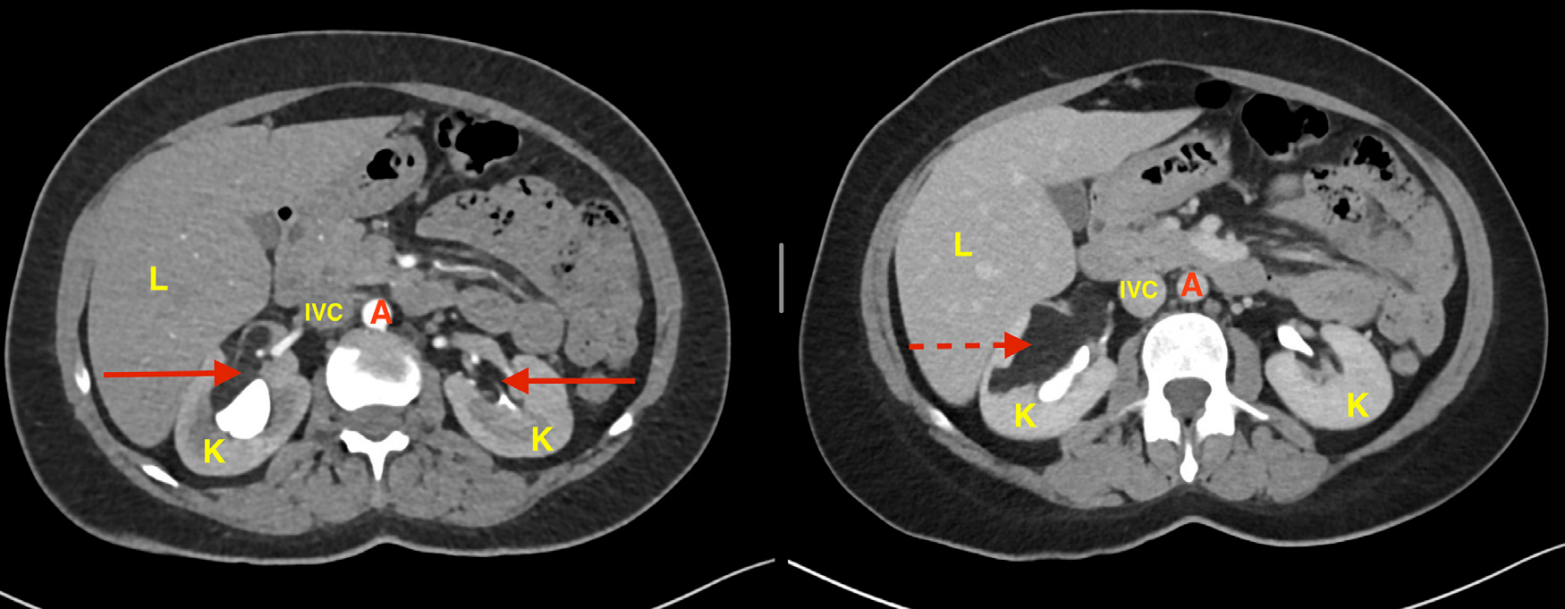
CT abdomen axial cut with contrast at 2 levels. • K: kidney. • L: liver. • A: abdominal aorta. • IVC: inferior vena cava. • Red solid line arrows demonstrating the right and left fat rich renal masses. • Red dotted line arrow showing the right renal mass involving the lateral cortex.
